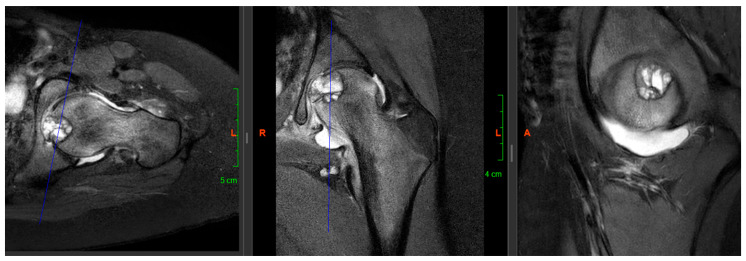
Preoperative MRI scan (transverse, coronal and axial sections).
